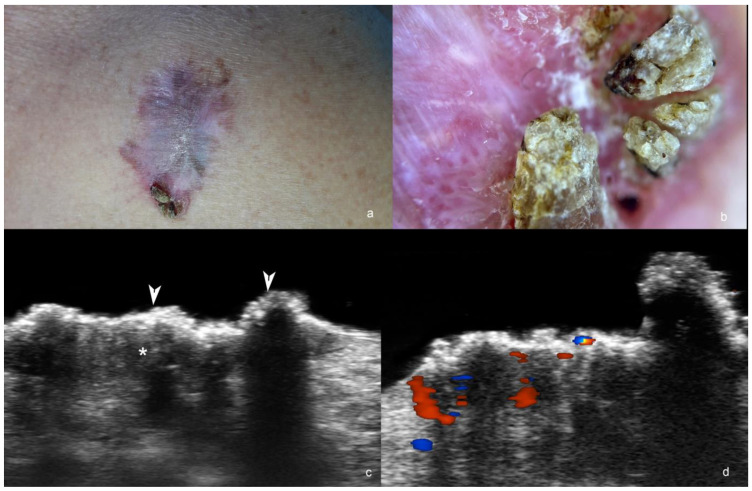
(a–d). Squamous cell carcinoma. (a). Clinical image of the lesion at the anterior aspect of the thorax. (b). Dermoscopy. (c,d) Ultrasound at 71 MHz ((c), greyscale; (d), color Doppler, transverse views) show ill-defined hypoechoic dermal structure (*) without hyperechoic spots and with crumpled, exophytic and irregular epidermis (arrowheads). On color Doppler (d), the lesion has increased vascularity.
